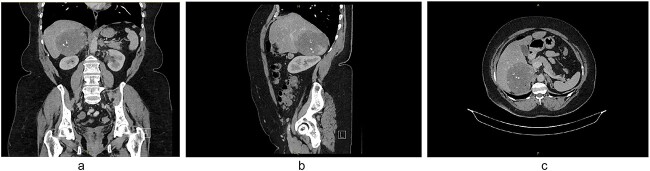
(a) CT coronal, (b) CT sagittal, (c) CT axial scans demonstrating a right-sided adrenal lesion (9.5 × 8.6 cm) with fat lobules, calcification and heterogeneous progressive enhancement.
